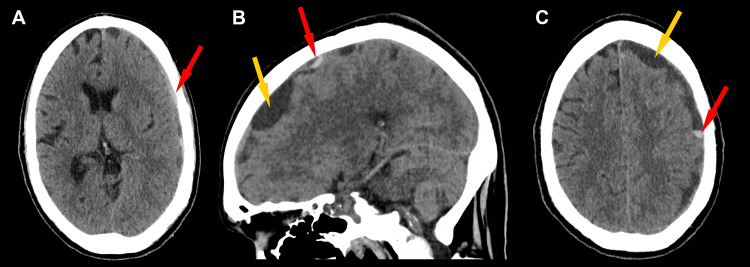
(A) Preoperative non-contrast CT of the head showing a left subdural hematoma with acute components (red arrows). (B and C) Non-contrast CT of the head, sagittal and axial views respectively, showing acute-on-chronic subdural hematoma (yellow arrows), with a notably loculated appearance due to chronic membrane formation. CT, computed tomography
